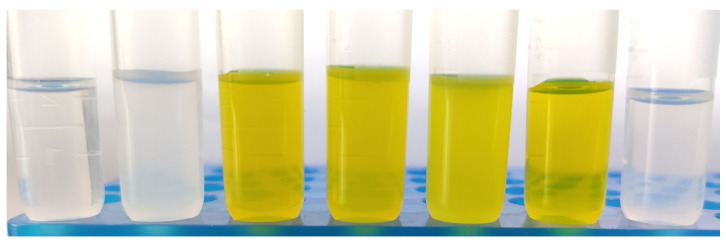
Optical photographs were taken for the appearance of liposomes. From left to right: Empty–DP–Lip, Empty–Lip, CT–DP–Lip, CT–TW80–Lip, CT–Lip, C–DP–Lip, and T–DP–Lip.
